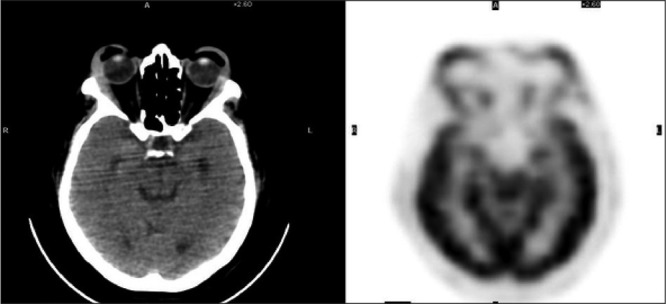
Axial CT and PET images of the orbita in the third PET/CT scan
